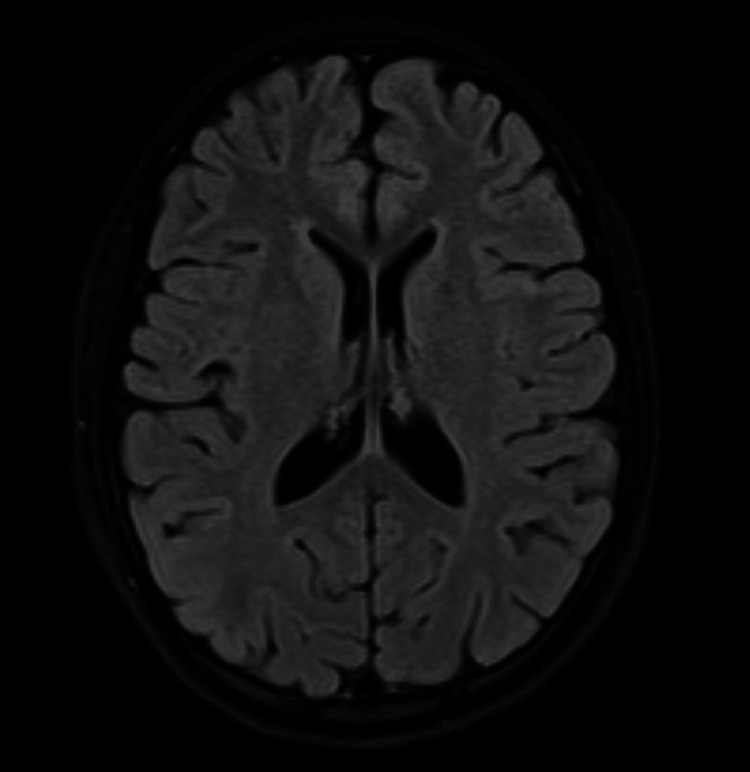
MRI brain (FLAIR) at the one-month follow-up shows resolution of the lesions in the basal ganglia and occipital region. FLAIR: fluid-attenuated inversion recovery
